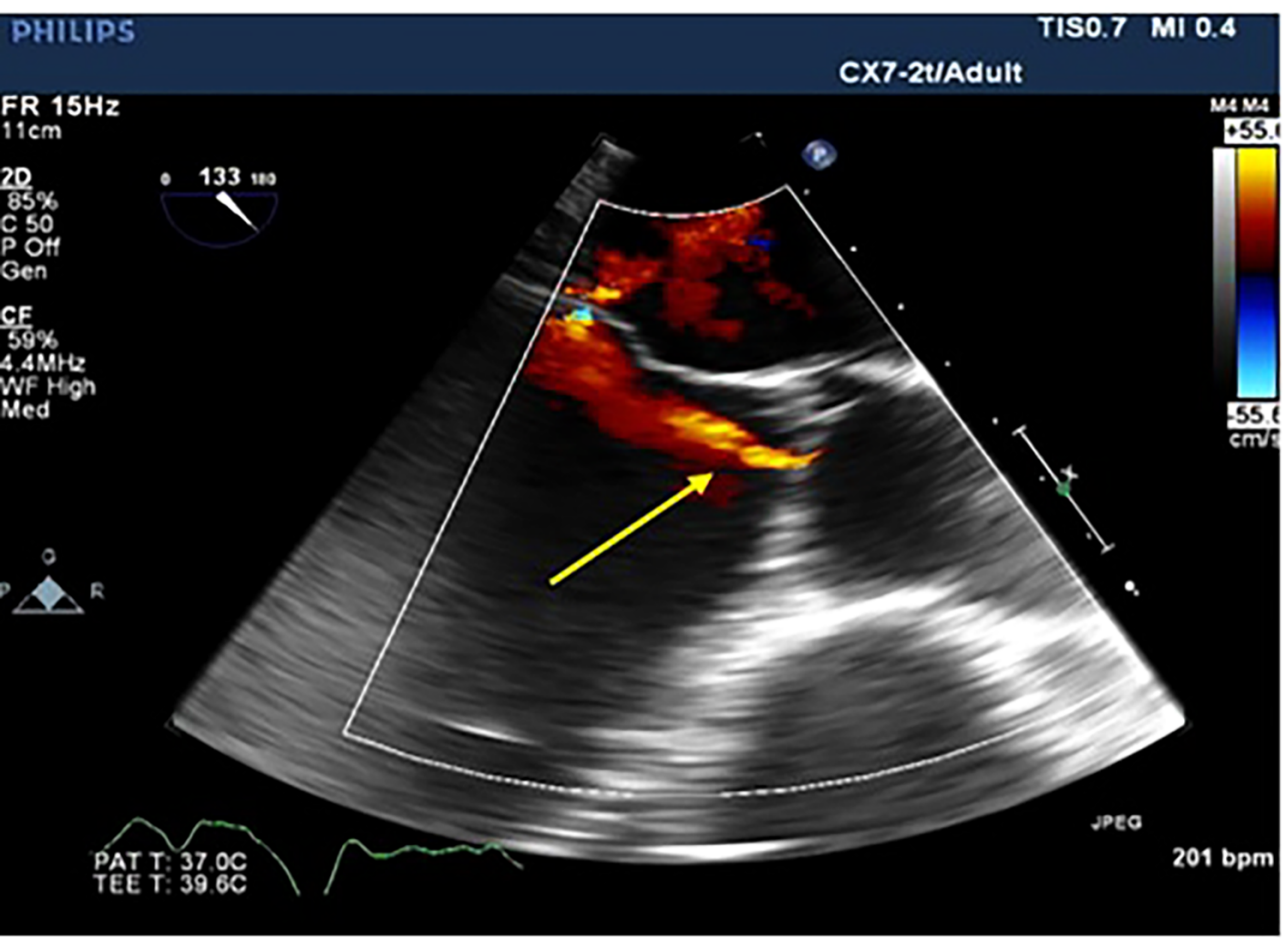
Transesophageal echocardiographic long access view of the aortic valve showing aortic regurgitation jet (yellow arrow).
